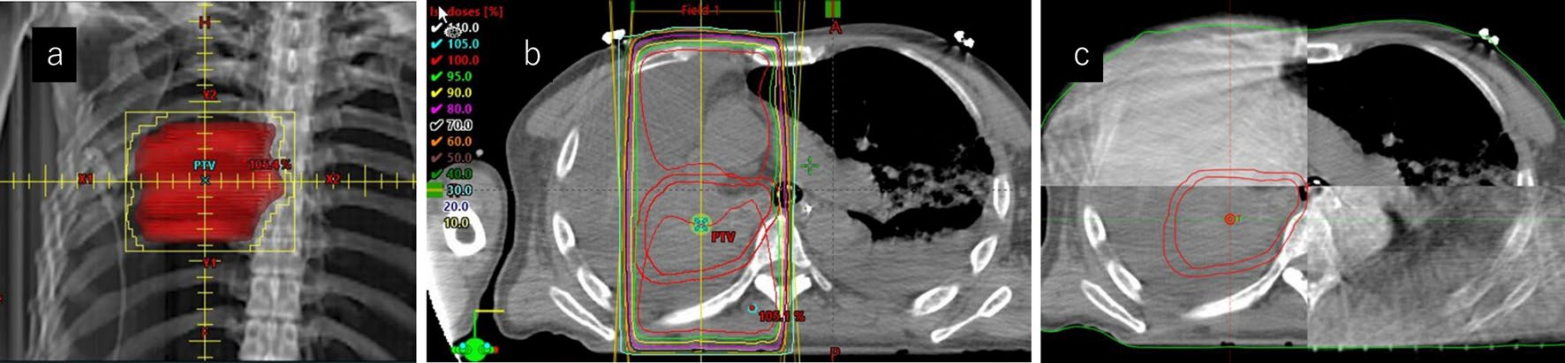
a–c Radiotherapy. a Planning target volume is red contouring area. b 3D-CRT planning. c Cone beam CT
